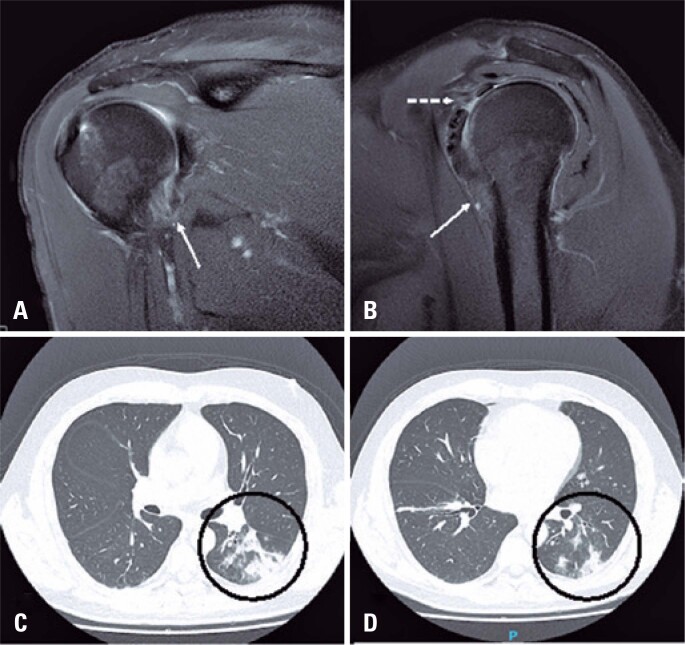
(A) A 48-year-old male patient submitted to magnetic resonance imaging study of the right shoulder with recent symptoms of pain and limited range of movement, almost simultaneously with diagnosis of COVID-19. Magnetic resonance imaging showed findings consistent with adhesive capsulitis, with capsular thickening (solid arrows in A and B) and pericapsular edema affecting the rotator interval (dashed arrow in B). During the same period, the patient was admitted to hospital due to shortness of breath and decreased oxygen saturation, and was diagnosed as COVID-19. A computed tomography scan of the chest revealed areas of ground glass opacities and alveolar opacification in the left lower lobe (circles in C and D)
